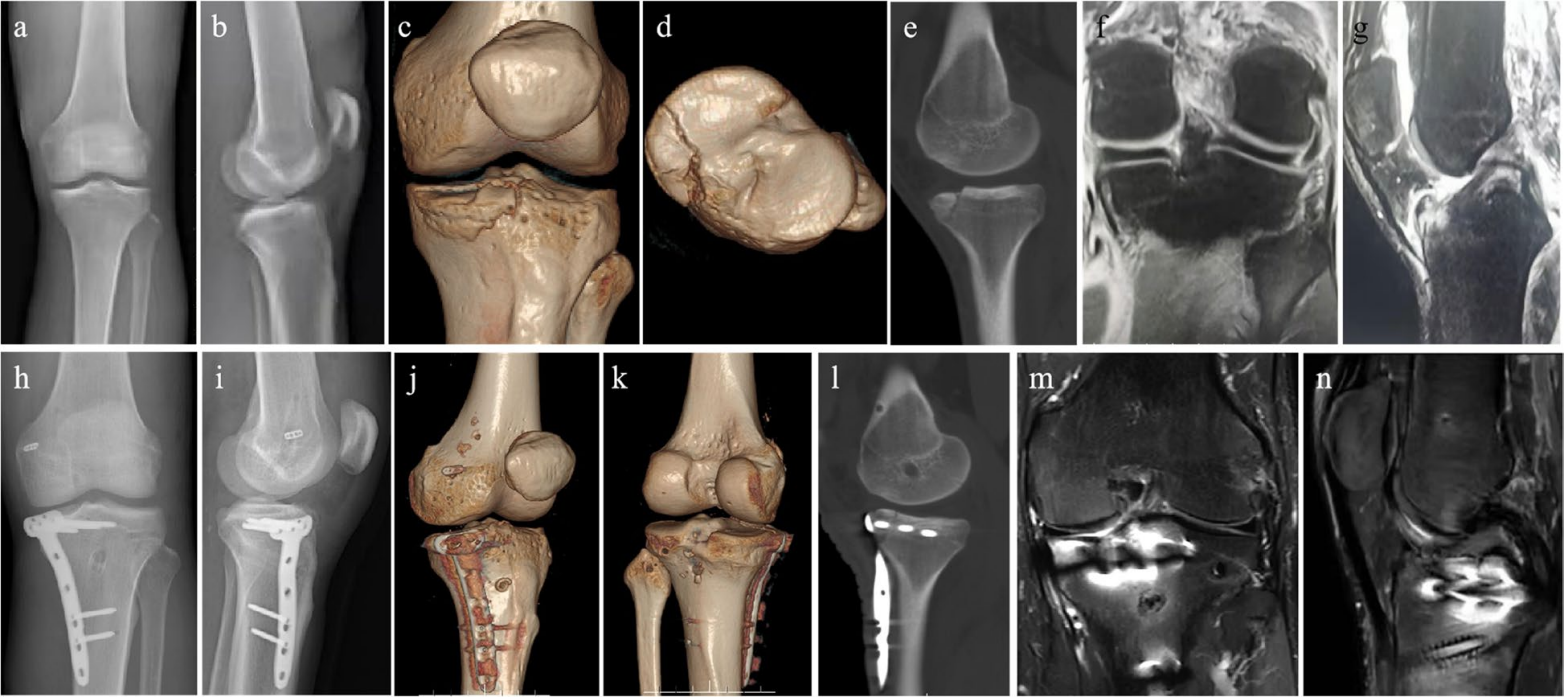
A 24-year-old man was injured in a fall during a basketball game (case 1). a-e Preoperative x-ray and CT images showed the anteromedial tibial plateau fracture. f-g Preoperative MRI images showed that the PLC and PLC injuries. h–l Postoperative X-ray and CT images showed an anatomical reduction of the tibial plateau fracture, and the PCL and PLC tunnels were in a good position. m–n MRI showed that the reconstructed ligament had good appearance and tension at the last follow-up
